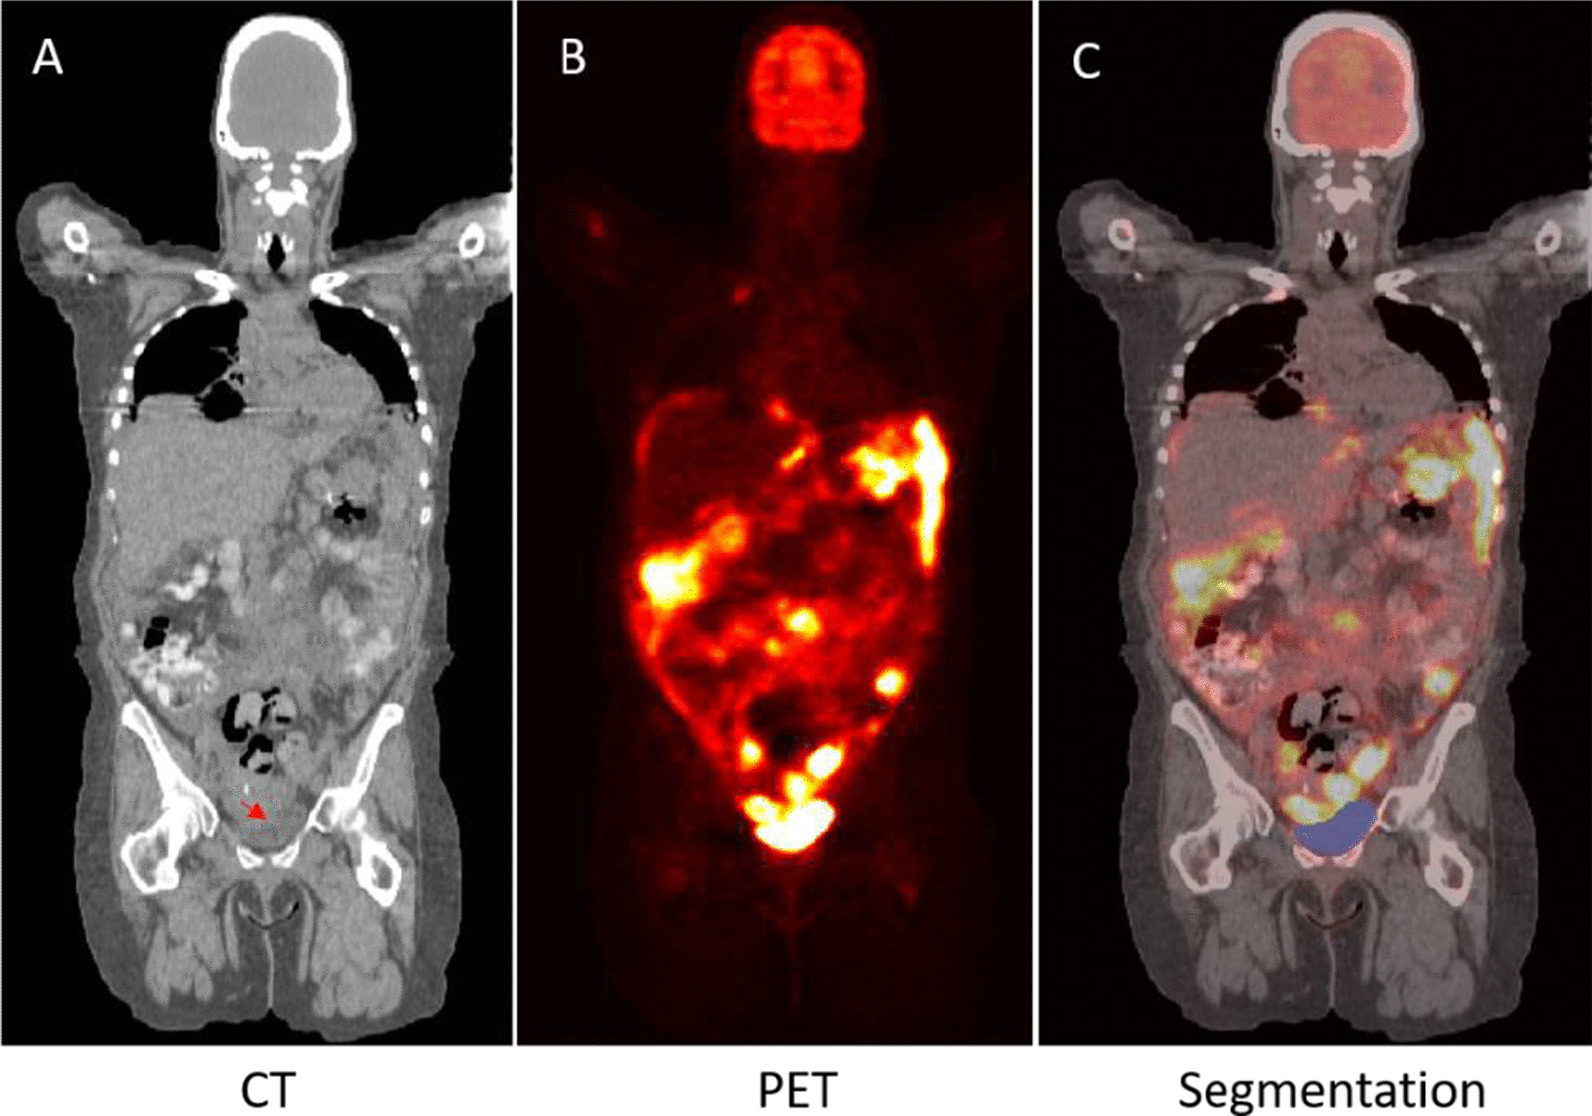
a CT image, b PET image, c segmentation by the model with mask overlaid in blue. The red arrow on CT image points out the bladder and tumor boundary
